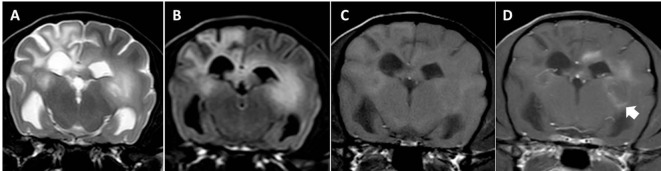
Transverse magnetic resonance images (3 T) of a Yorkshire Terrier (2 years, female) with necrotizing leukoencephalitis. T2 (A) and fluid-attenuated inversion recovery (B) hyperintense finger-like lesions affecting the subcortical white matter, the corona radiata, and the diencephalon. Right-sided ventricular enlargement, focal widening of sulci, and mild midline shift to the right are most likely secondary to white matter loss in the right prosencephalon. Contrast enhancing lesions as on the left side [(C) T1-weighted native; (D) T1 weighted after contrast injection] and non-contrast enhancing lesions (right side) may coexist in one patient, which most likely reflects different stages of the disease. Enhancement may be patchy or it may be seen around necrotic areas (ring-like, arrow head). Meningeal contrast enhancement is deficient.
